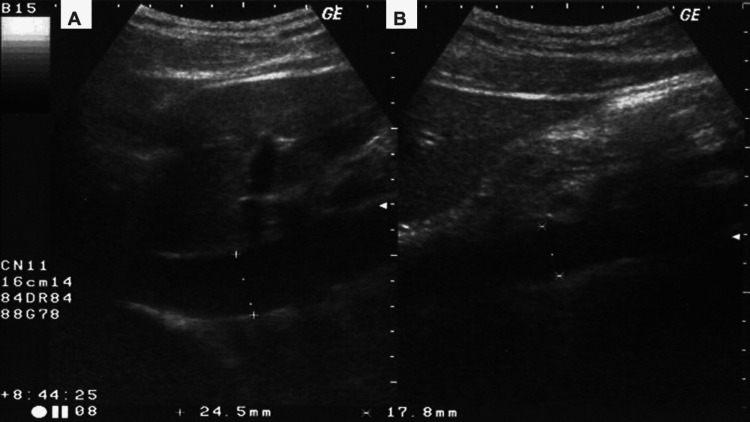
Ultrasound measurement point location for eIVC and aorta A: Expiration IVC; B: Systole aorta eIVC: Expiration Inferior Vena Cava
